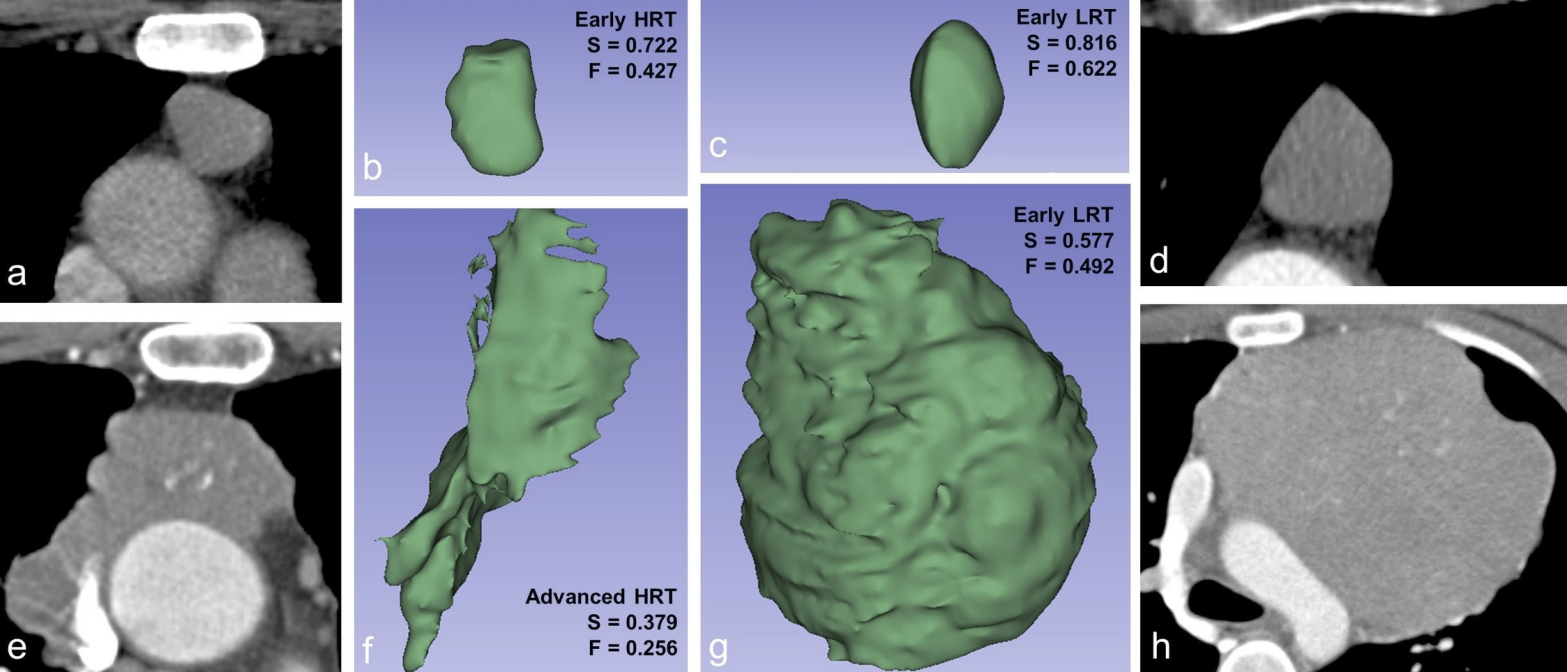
Axial CT slices (a, d, e, h) and corresponding volume renderings (b, c, f, g) of segmented VOIs with calculated values for sphericity (S) and flatness (F). LRT: Low-risk thymic epithelial tumor. HRT: High-risk thymic epithelial tumor.
